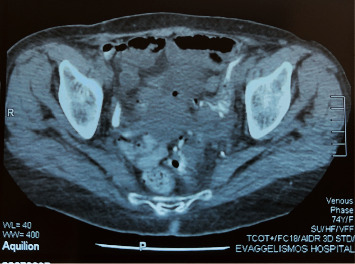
CT scan showing findings of chronic diverticulitis.
